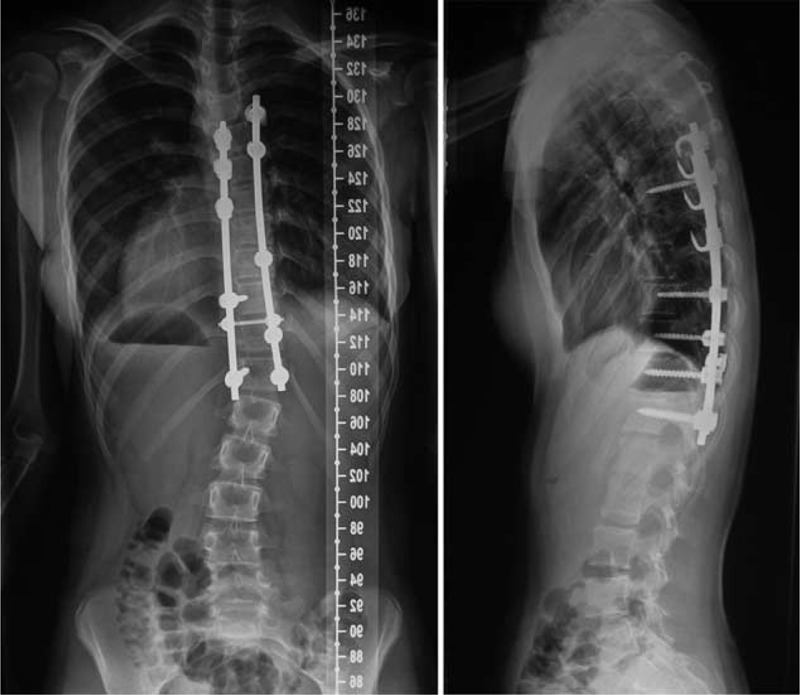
Standing anteroposterior and lateral radiographs of 24 months after reoperation.
